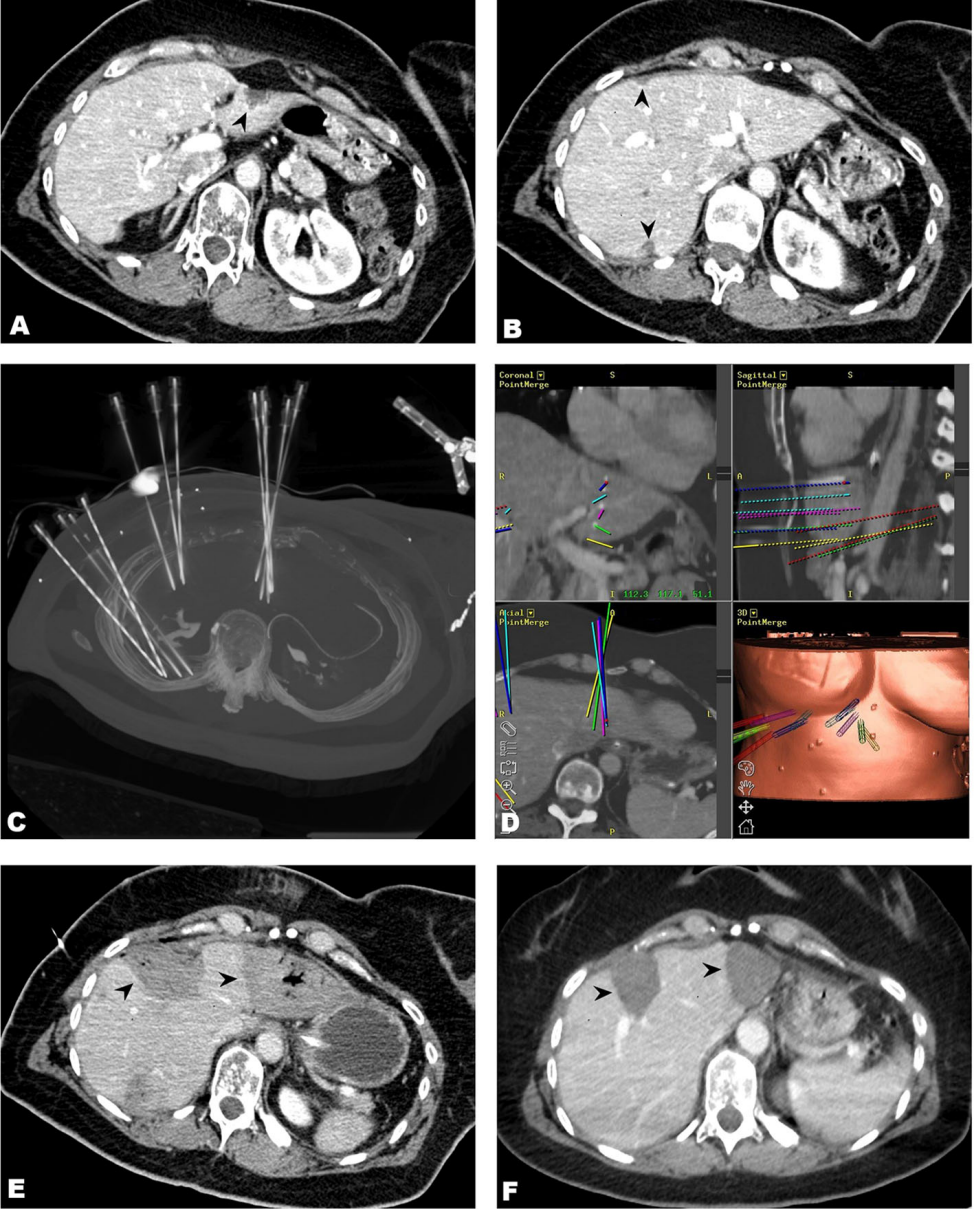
A 50-year-old female with breast cancer liver metastases measuring up to 3.0 cm. A Contrast enhanced CT-image of one BCLM (black arrowhead) in segment II/III close to the stomach. B Contrast enhanced CT-image showing the other two subcapsular BCLMs in the right lobe (black arrowhead). C Maximum Intensity Projection image of the control CT with 12 coaxial needles in place. D Fused images of the control CT with the planning CT showing superposition of planned paths with inserted coaxial needles. E, F Black arrowheads demonstrating ablation zones on CT images immediately after the procedure and after 36 months later without evidence of local recurrence
